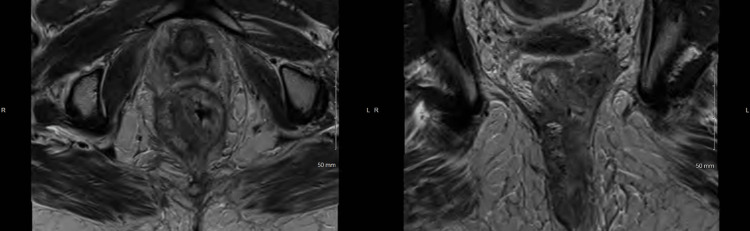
Pelvic MRI showing a locally advanced recurrent anal cancer involving the left pelvic sidewall in the axial (left) and coronal (right) views for case 1
